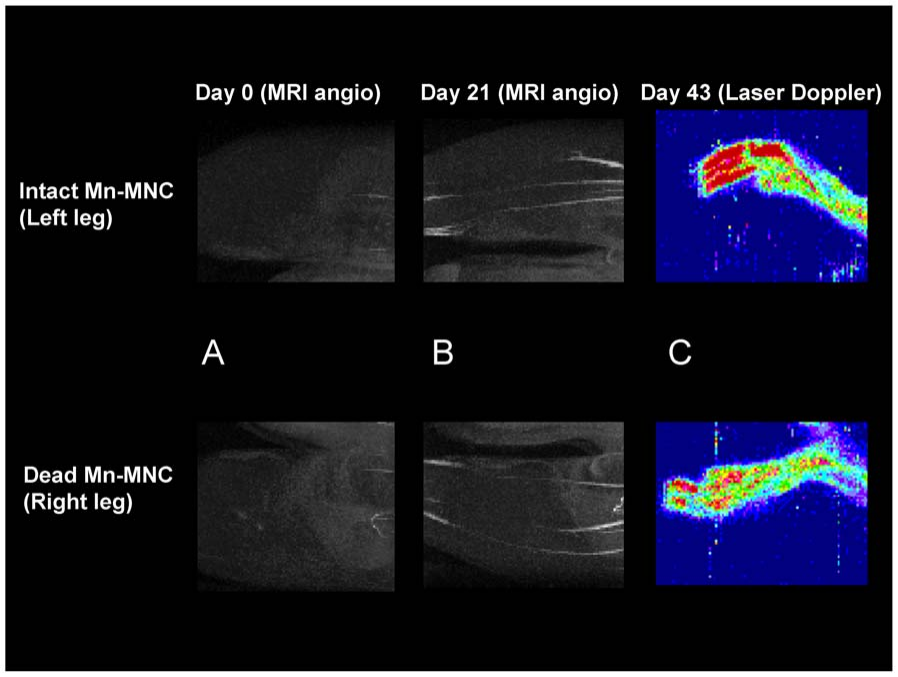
Chronic evaluation of angiogenesis using MRI angiography and Laser Doppler perfusion imaging. Representative MRI angiographs (A and B) and laser Doppler perfusion images (C) are presented. The MRI angiographic images were obtained at 0 (A) and 21 (B) days after muscle ischemia and MNC transplantation. Recovery of arterial blood flow was observed in the hindpaw for both the intact and dead MNC administered legs at 21 days. The intact MNC transplanted side showed a similar number of arteries to the contralateral side containing dead MNCs. The laser-Doppler perfusion image was acquired 43 days after the ischemia and transplantation (C). The muscle perfusion measured with laser-Doppler in the left legs (injected with intact MNCs) was 20±9% (n = 6) higher than that in the right legs (dead MNCs).
